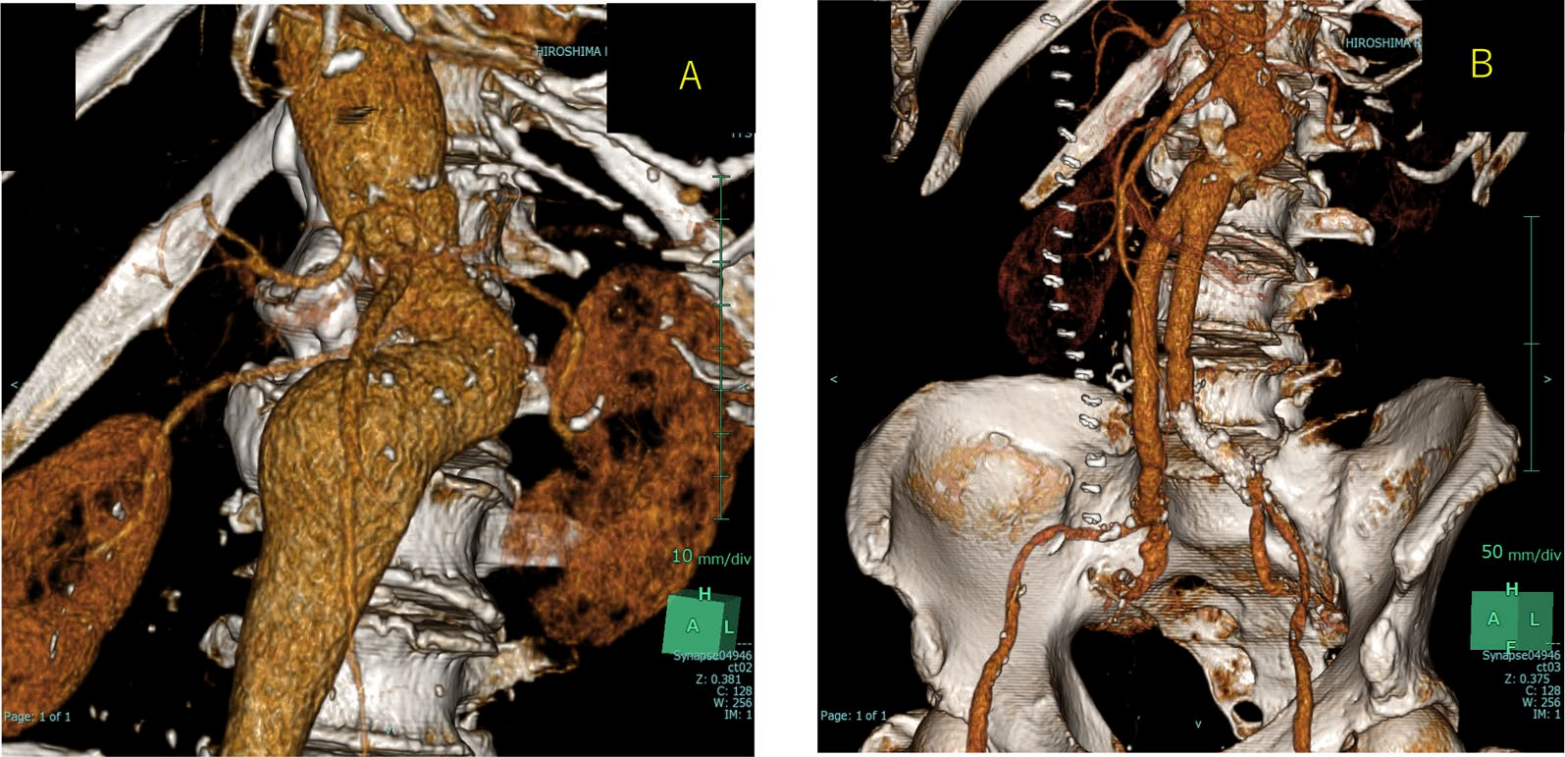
A Preoperative three-dimensional computed tomography (CT) image showing the severe proximal neck of the AAA. B Postoperative three-dimensional CT image showing the exclusion of the aneurysm and the preservation of the bilateral renal arteries in the patent graft
